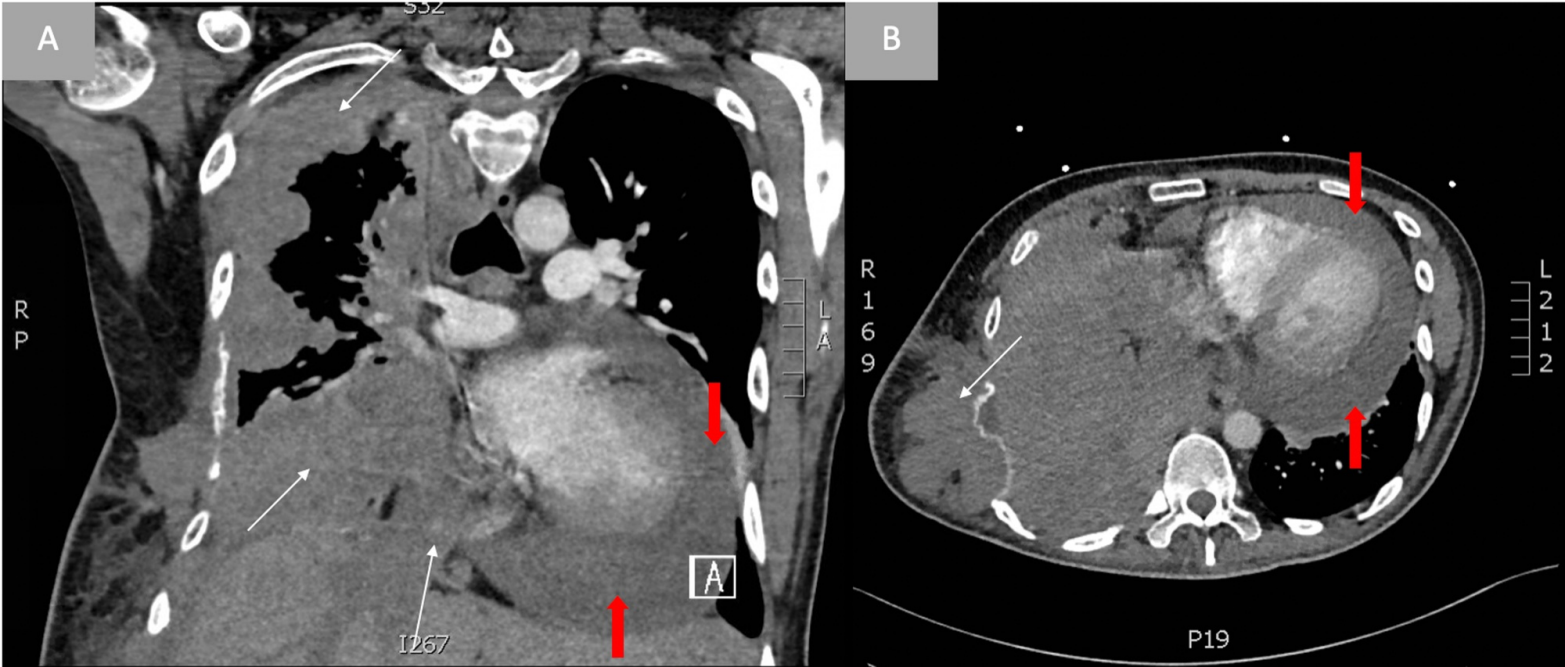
CT findings representing metastatic foci of disease to lung and epicardium (white arrows) as well as pericardial effusion (red arrows) with coronal view (A) and transverse view (B)
